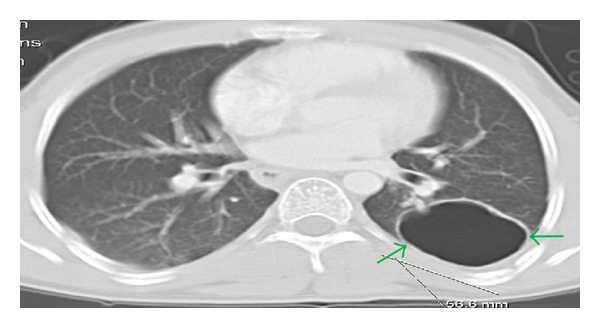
Chest CT: residual 6 cm thin walled cyst in the posterior aspect of the left midlung field (green arrows).
